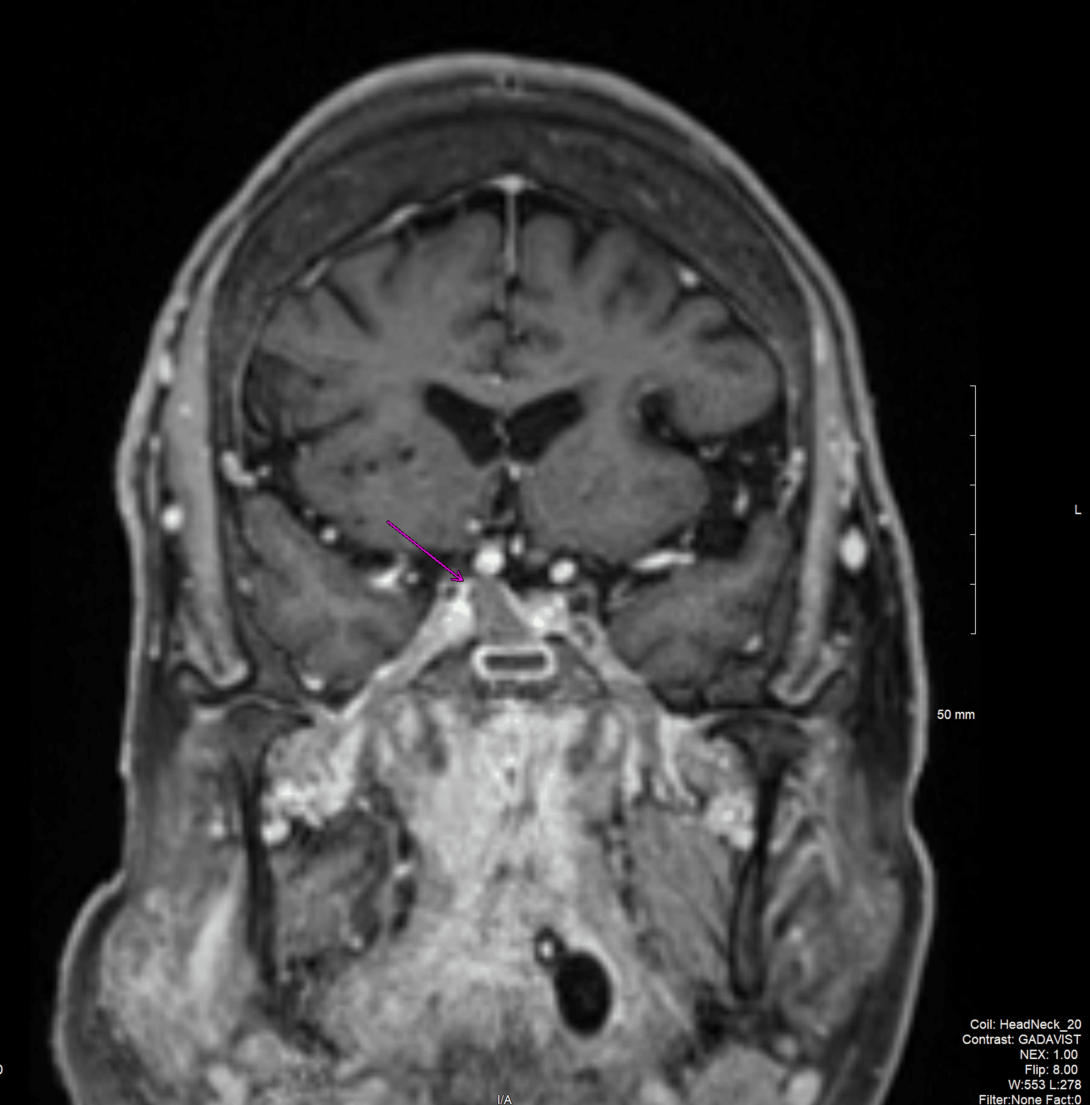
Coronal CT showing enlarged sella with asymmetrical density on the right side (pink arrow), which may represent a pituitary adenoma
